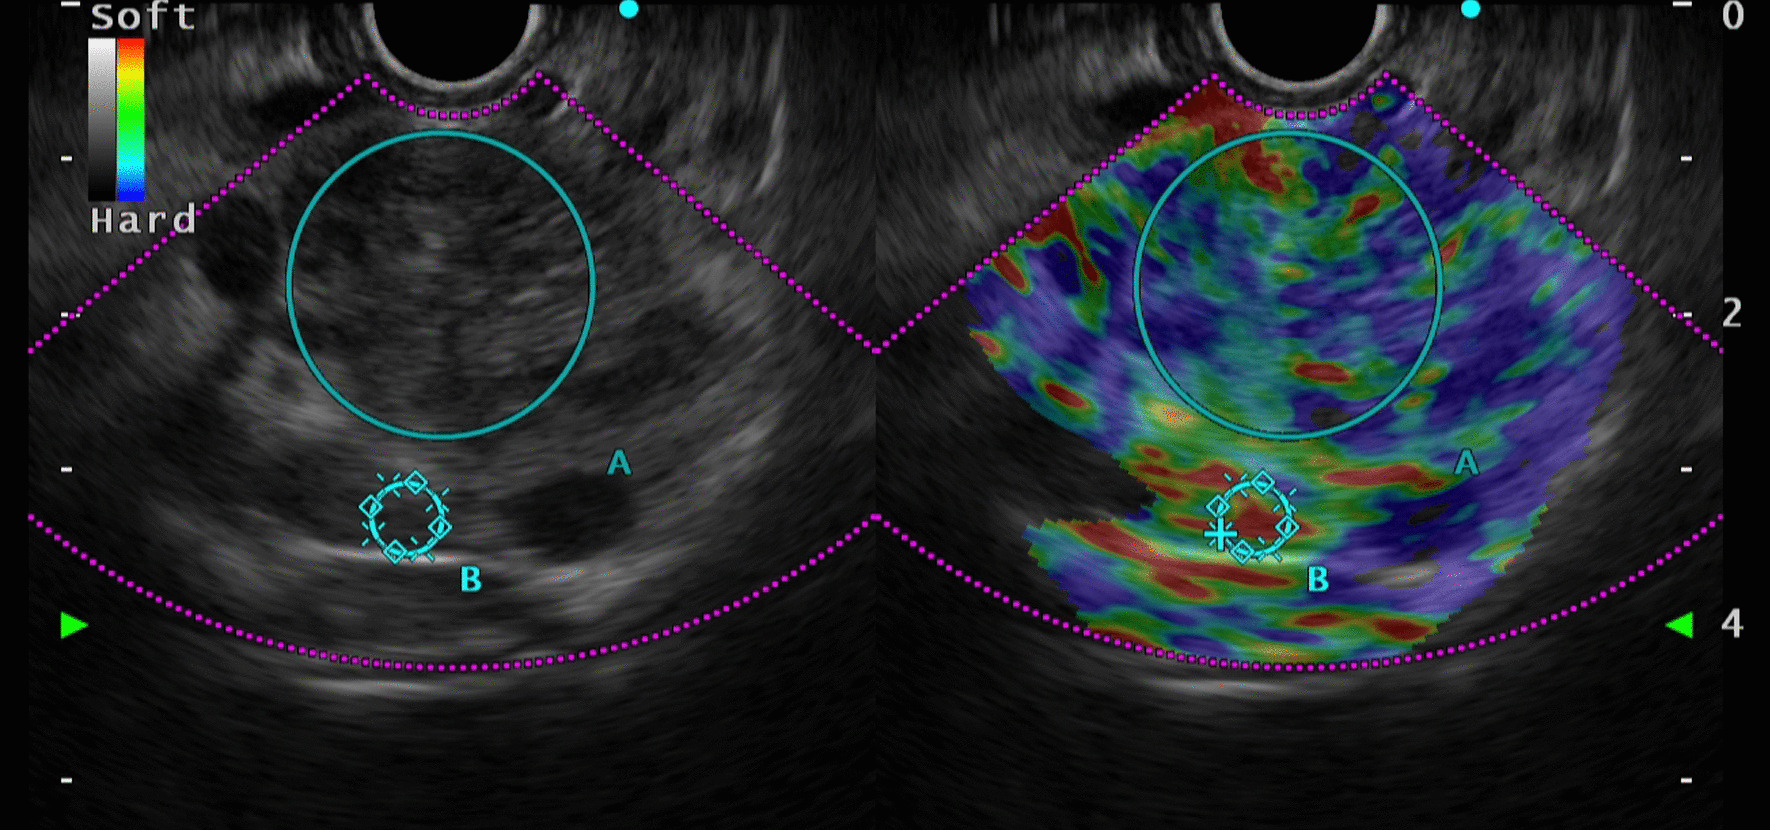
Endoscopic ultrasound study demonstrated a hypoechoic lesion measuring 43 × 35 mm^2^ lying at the posterior wall of the stomach. Tumor encasement of the left gastric artery and a short segment of celiac trunk without obstruction was detected. Elastography strain ratio of the lesion was estimated to be 22
